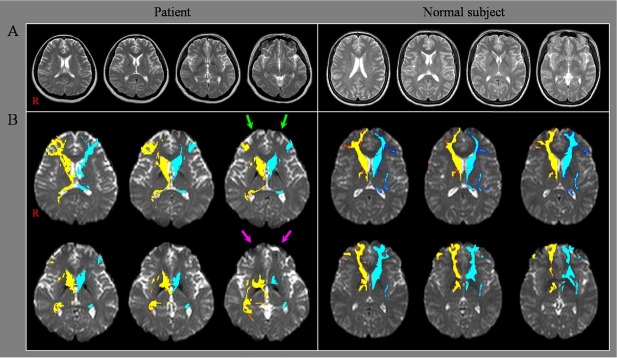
(A) T2-weighted brain MR images at seven months after onset show no abnormality. (B) The neural connectivities of the caudate nucleus to Brodmann areas 10 and 12 (green arrows, medial prefrontal cortex) and Brodmann areas 11 and 13 (purple arrows, orbitofrontal cortex) in both hemispheres are decreased compared to a normal subject (40 year-old female).
